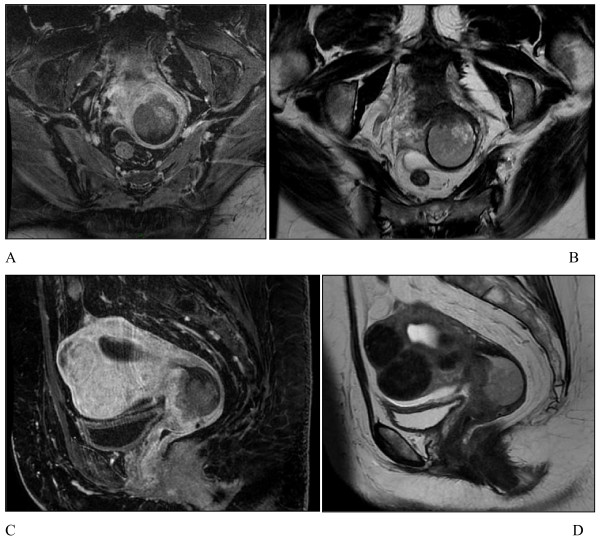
MRI findings. Mid-sagittal pelvic (A) T1-weighted post gadolinium and (B) T2-weighted images, showing an endocervical tumor with necrotic areas and large typical uterine interstitials fibroids. Axial pelvic (C) T1-weighted post gadolinium and (D) T2-weighted images at the tumor level.
